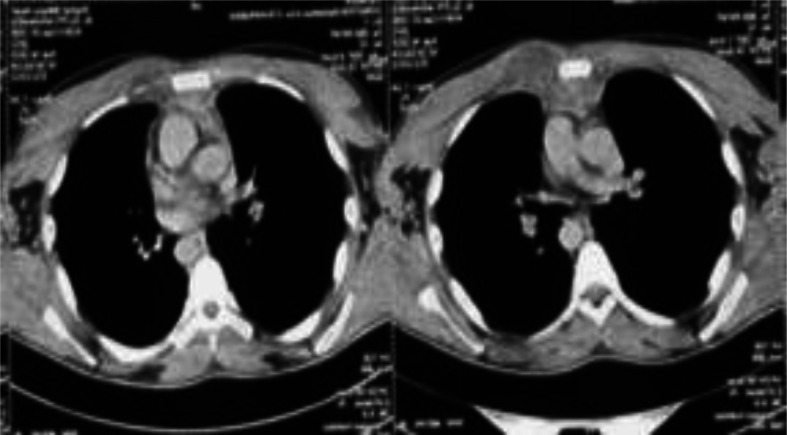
Thoracic CT scan showing a pre- and retrosternal collection extending towards the cervicothoracic orifice, measuring 63×74×97 mm, with sternal erosion and the onset of anterior mediastinitis.
